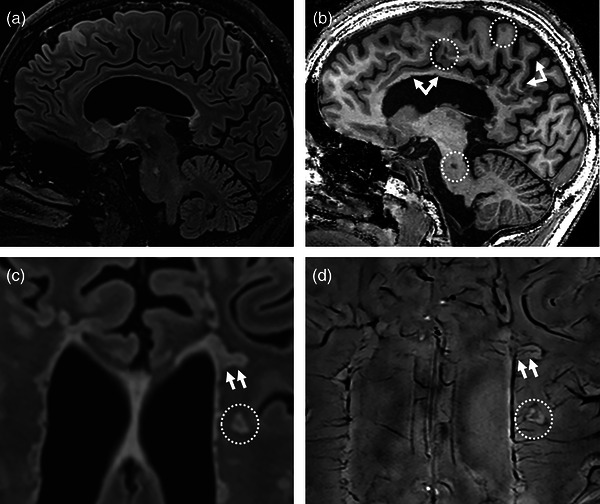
Ultra–high‐field MRI features of progressive multiple sclerosis. 7 T MRI of a 34‐year‐old individual with MS (symptom onset at age 26, diagnosis at age 27) who developed a progressive disease course at age 32, despite initiation of highly effective monoclonal antibody therapy at diagnosis and subsequent hematopoietic stem cell transplantation at age 28. Notably, no new focal lesions appeared after treatment initiation. FLAIR (a) and T1‐weighted (b) images demonstrate several features characteristic of progressive MS, including numerous cortical lesions and lesions in anatomically critical locations (white dashed circles in b), as well as diffuse thinning of the corpus callosum and cerebral cortex (white arrows in b). Higher‐magnification views of periventricular white matter (c, FLAIR; d, susceptibility‐weighted imaging) reveal lesions with paramagnetic rims (dashed circles in c and d), many of which are centered on a central vein (white arrows in c and d).
